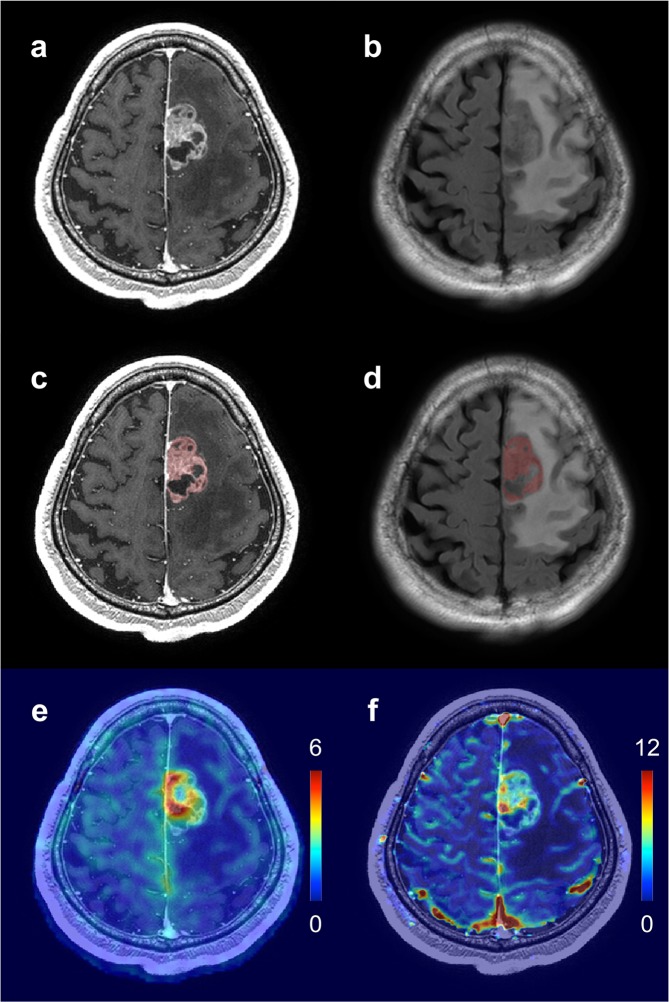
Example of tumor segmentation for a patient with WHO grade IV glioblastoma (59-year-old male). (a) CE-T_1_WI. (b) T_2_ FLAIR image. (c) The segmented tumor volume (*red color*) overlaid on the CE-T_1_WI. (d) The segmented tumor volume (*red color*) overlaid on the T_2_ FLAIR image. (e) The ASL-nCBF map overlaid on the CE-T_1_WI. (f) The DSC-nrCBF map overlaid on the CE-T_1_WI. WHO, World Health Organization; CE-T_1_WI, contrast-enhanced T_1_-weighted image; FLAIR, fluid-attenuated inversion recovery; ASL-nCBF, arterial spin labeling normalized cerebral blood flow; DSC-nrCBF, dynamic susceptibility contrast normalized relative cerebral blood flow.
